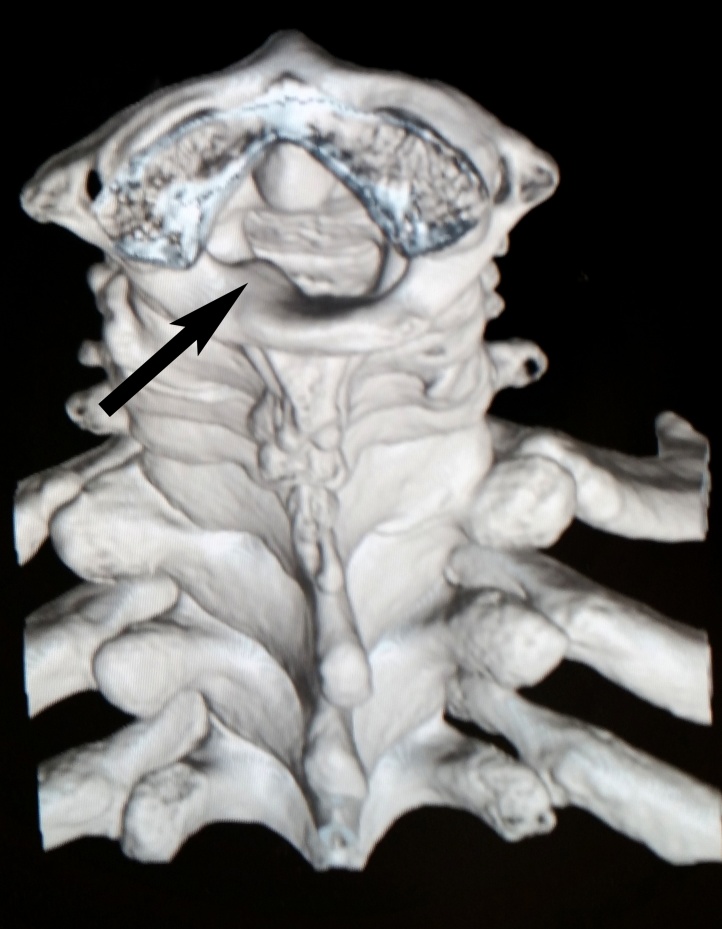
Cervical spine CT-scan showing expansile bone exostosis arising from the left lamina of C2 vertebra.
